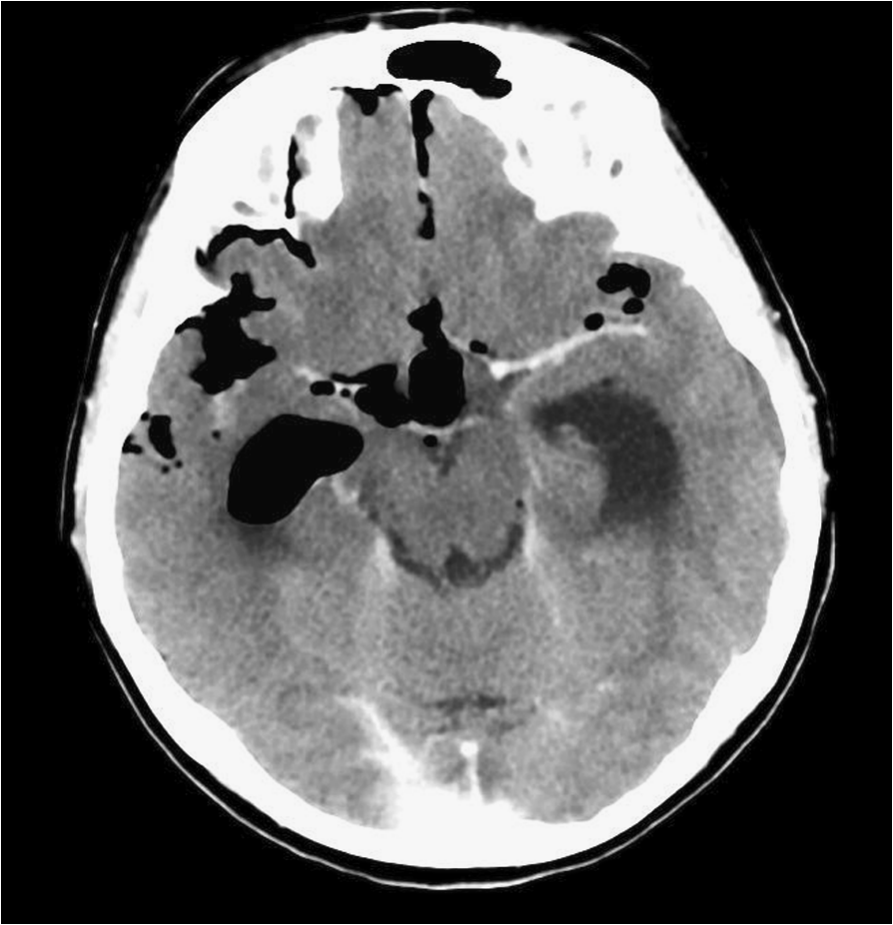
Computed tomography scan showing presence of air in the subarachnoid spaces and ventricles.
